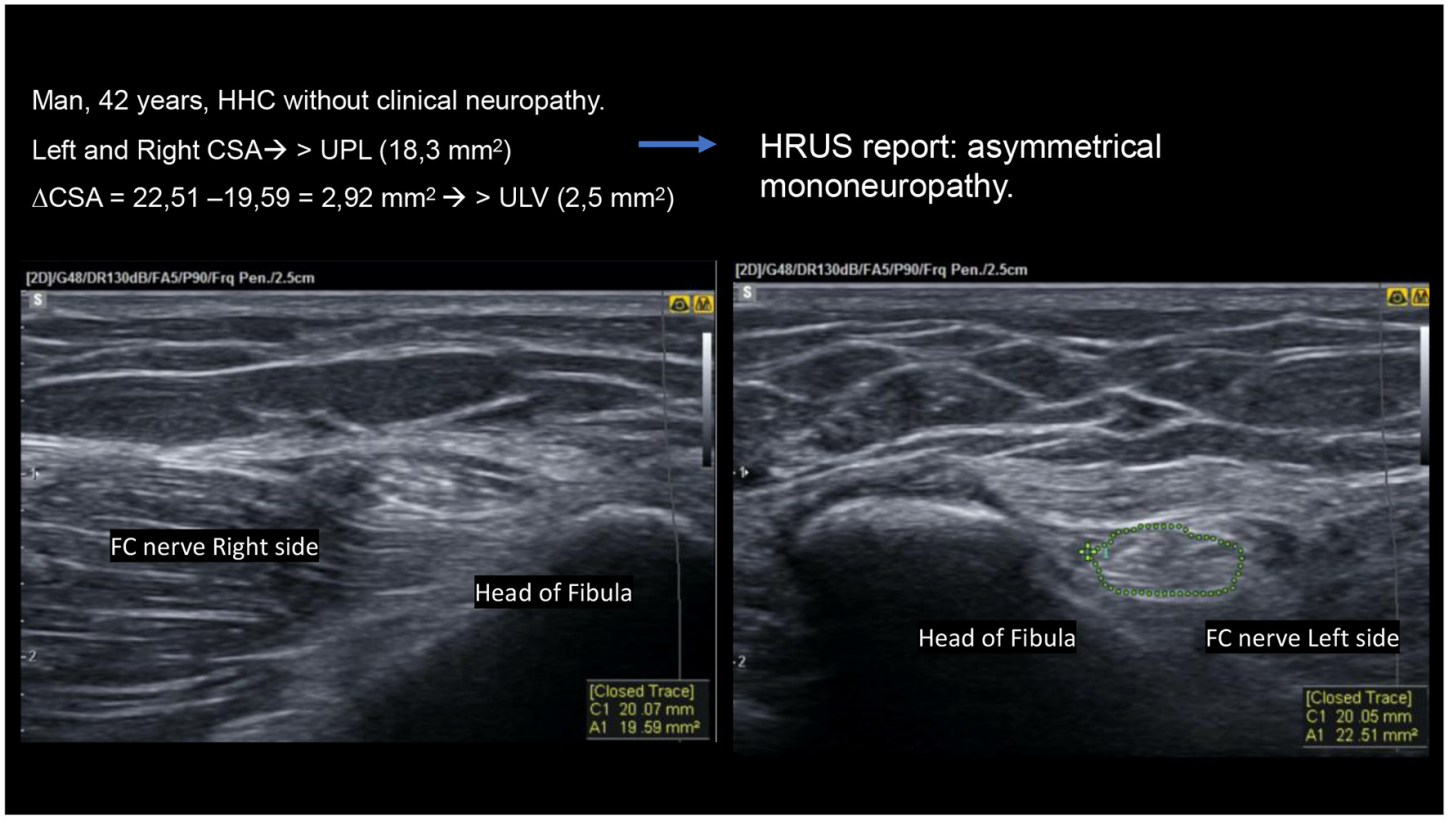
High-resolution ultrasound (HRUS) images of the same HHC of a patient with HD with asymmetrical mononeuropathy of the common fibular nerve on the head of the fibula.
